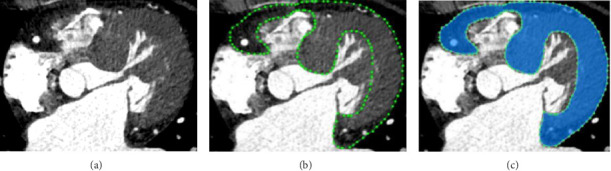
Professional software was used to delineate the structure of the interventricular septal-free wall. (a) HCM CT data; (b) Labelme software mark the target structure; (c) the structure of the interventricular septal-free wall.
